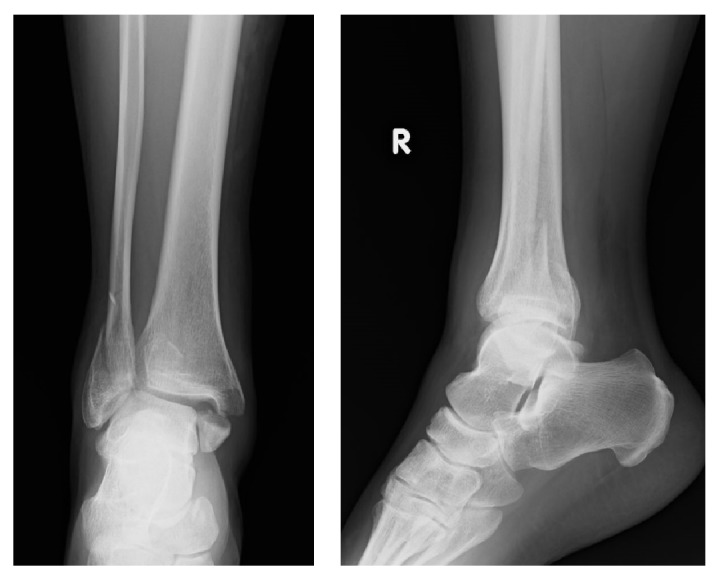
Initial radiographs at emergency hospital. Weber type B and LH classification's SE type stage 4 fractures.
